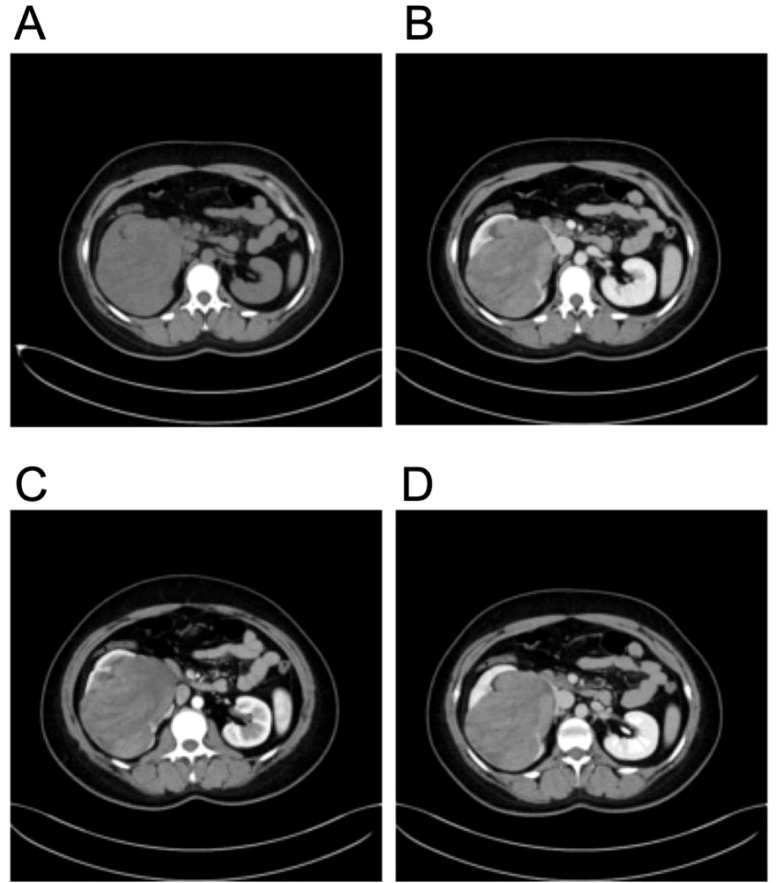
Representative contrast-enhanced computed tomography images of the large right renal mass in a 23-year-old female. (A) Axial non-contrast phase: A heterogeneous soft tissue mass measuring approximately 11.2 × 8.4 cm occupies a substantial portion of the right kidney, with no evidence of calcification, liquefaction, or obvious necrosis. (B) Axial corticomedullary/arterial phase: The mass demonstrates moderate and uneven enhancement, most prominent in the peripheral and septated-like internal components, with relative hypoattenuation in central areas. (C) Axial venous/portal phase: Persistent heterogeneous enhancement is seen throughout the mass, with continued uneven distribution and no washout pattern suggestive of simple cyst. (D) Coronal reformatted view: The mass causes significant mass effect on the kidney, with compression and displacement of the renal parenchyma, particularly evident in the lower pole and medial aspect, and extension toward the renal hilum/pelvis.
